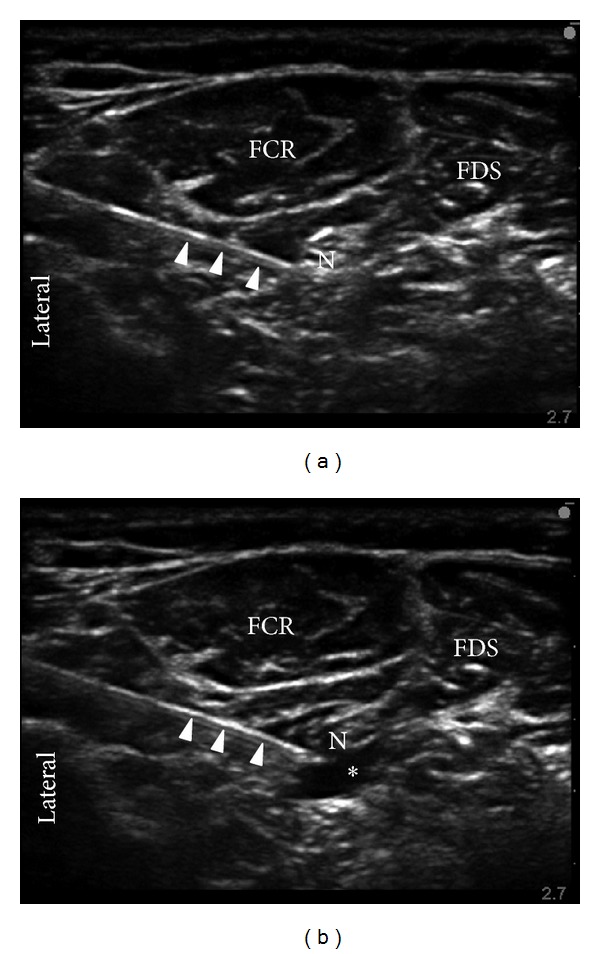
Short-axis sonograms of ultrasound-guided median nerve block at two time points: preinjection (a) and postinjection (b); arrows identify the needle; N = nerve; FCR = flexor carpi radialis muscle; FDS = flexor digitorum superficialis muscle; ∗ = injectate solution.
